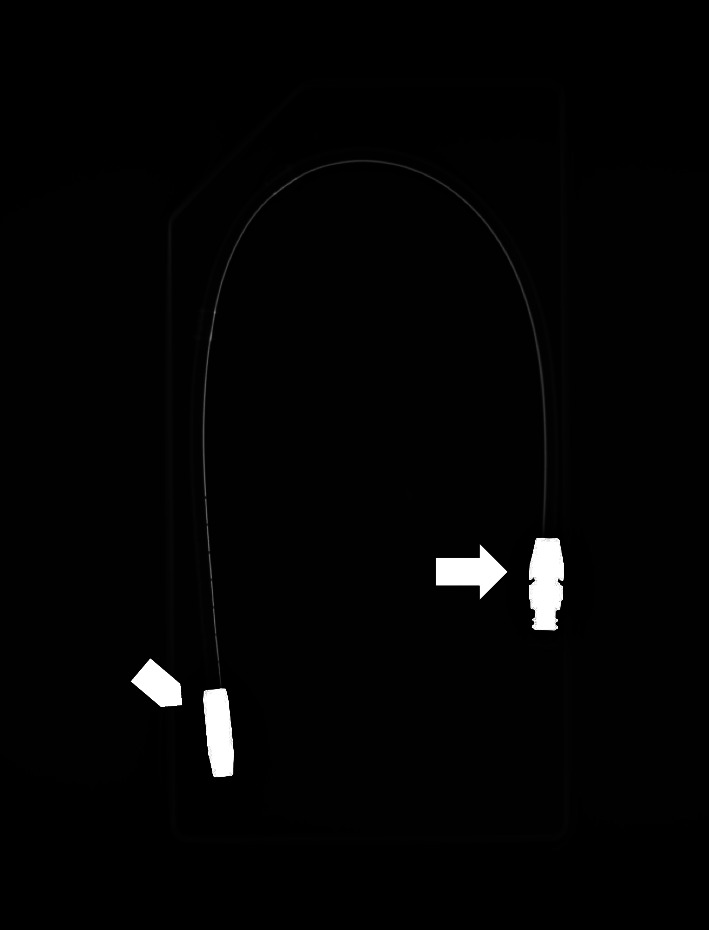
Conventional X-ray of the peritoneal dialysis catheter that demonstrates the radiopaque tip tungsten ballast (arrowhead) and, on the other extremity, the titanium connector (arrow).
